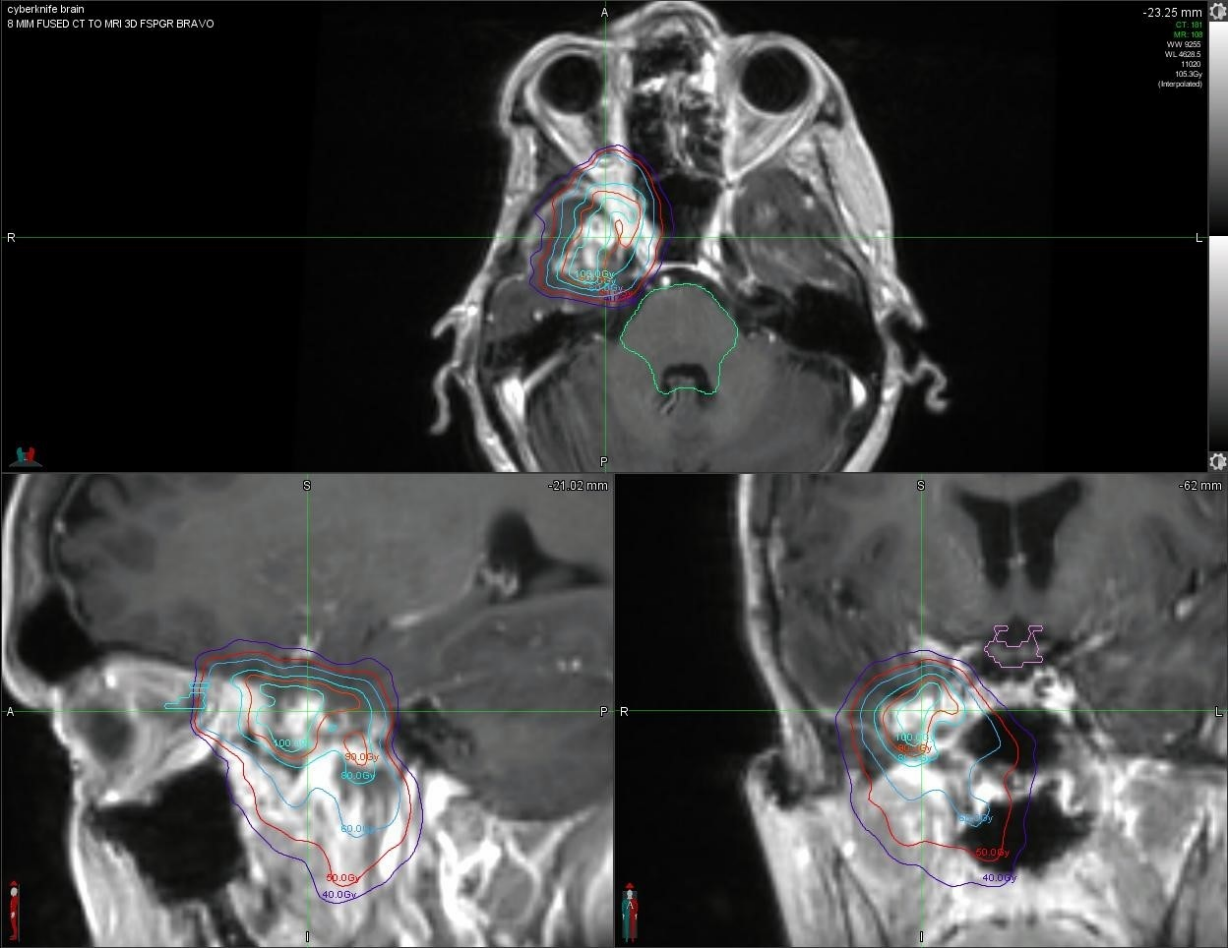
The computed tomography and the magnetic resonance imaging fusion of the orbital metastasis.
